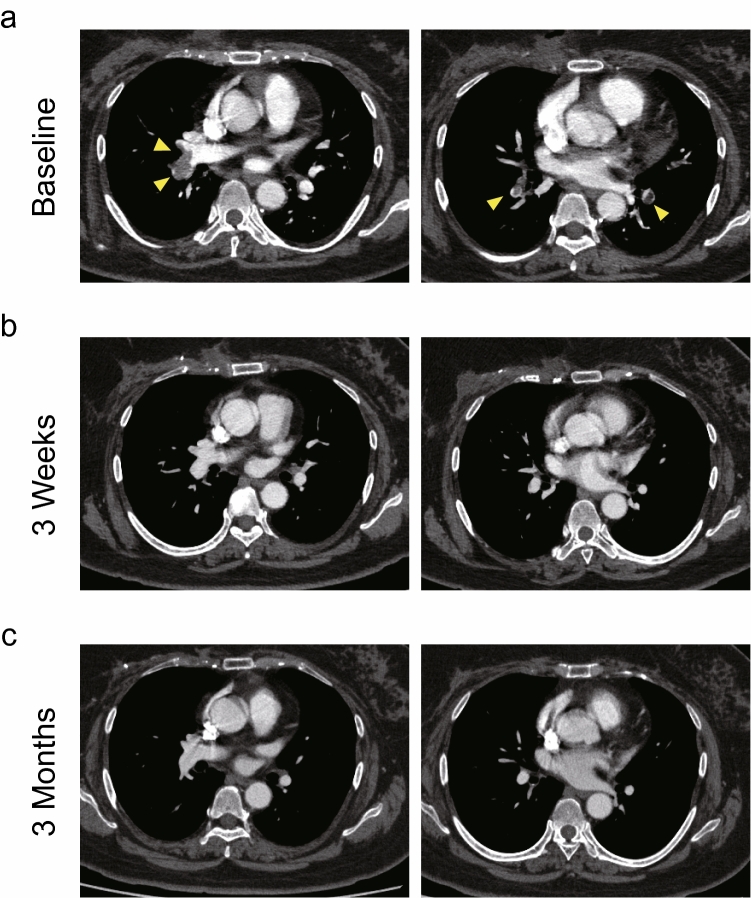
Representative CT scan images of pulomonry thromboembolism in patients with breast cancer who received rivaroxaban treatment. (a) Bilateral pulmonary thromboembolism were ditected by contrast-enhanced CT scan at baseline. Yellow arrows show thrombi in pulmonary artery. (b) After the treatment with rivaroxaban 30 mg for 3 weeks, pulmonary thrombi were disappeared, achieving complete thrombus resolution. (c) There were no venous thromboembolism reccurence after the treatment with rivaroxaban 15 mg for 3 months.
